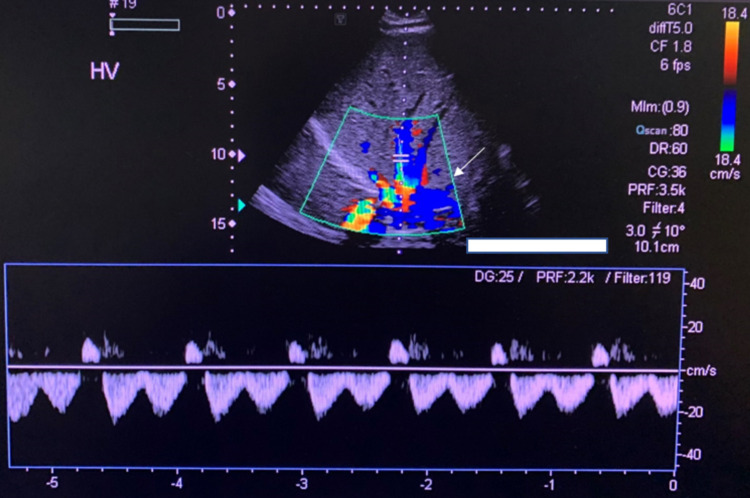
Ultrasound with Doppler of the hepatic vein. Arrow points to the hepatic vein which revealed a normal flow and direction of blood on doppler
